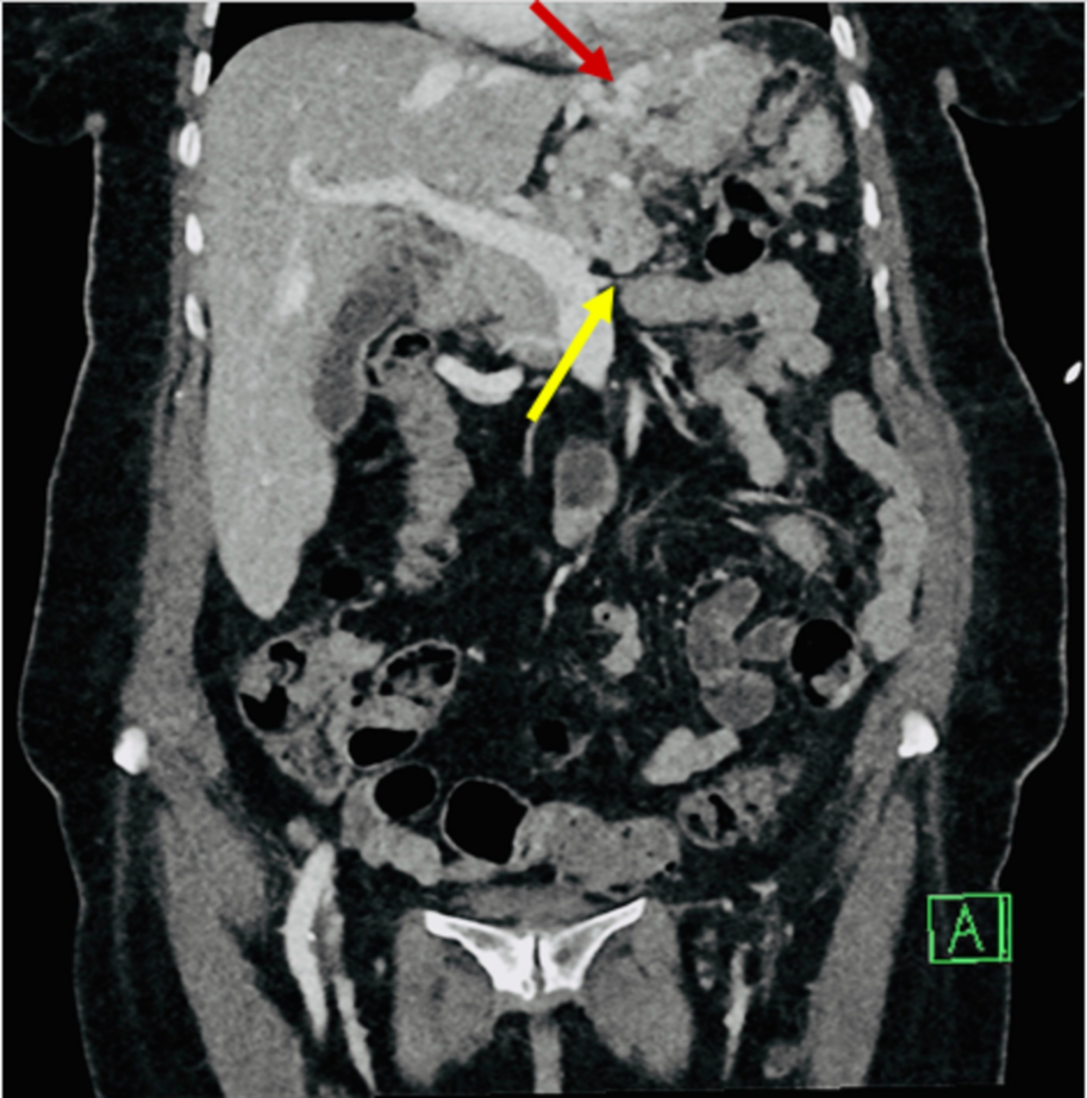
Coronal abdominal computed tomography (CT) scan Portovenous delay showing gastric fundal varies (red arrow) and termination of splenic vein circulation (yellow arrow) secondary to thrombosis
